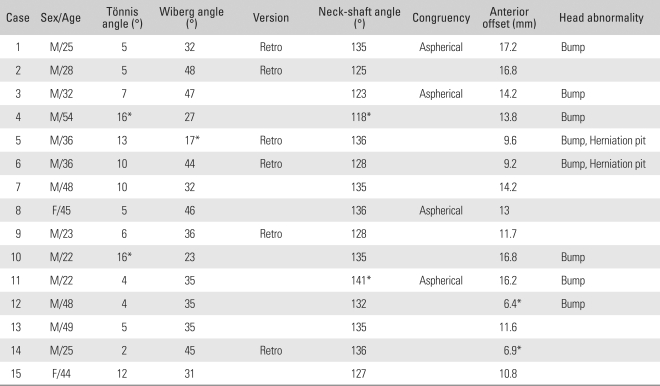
Results of the Radiographic Assessments
